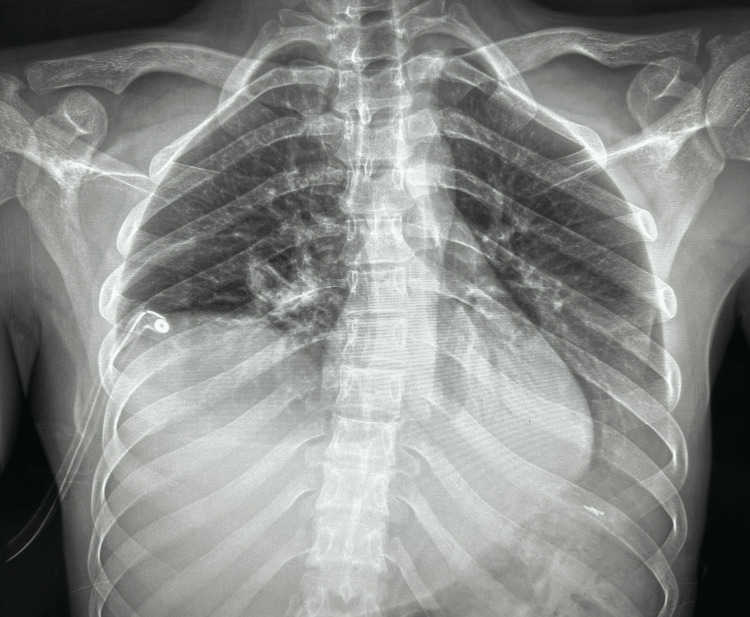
Postoperative chest X-ray after correction of the diaphragmatic defect showing a full expansion of the left lung.
